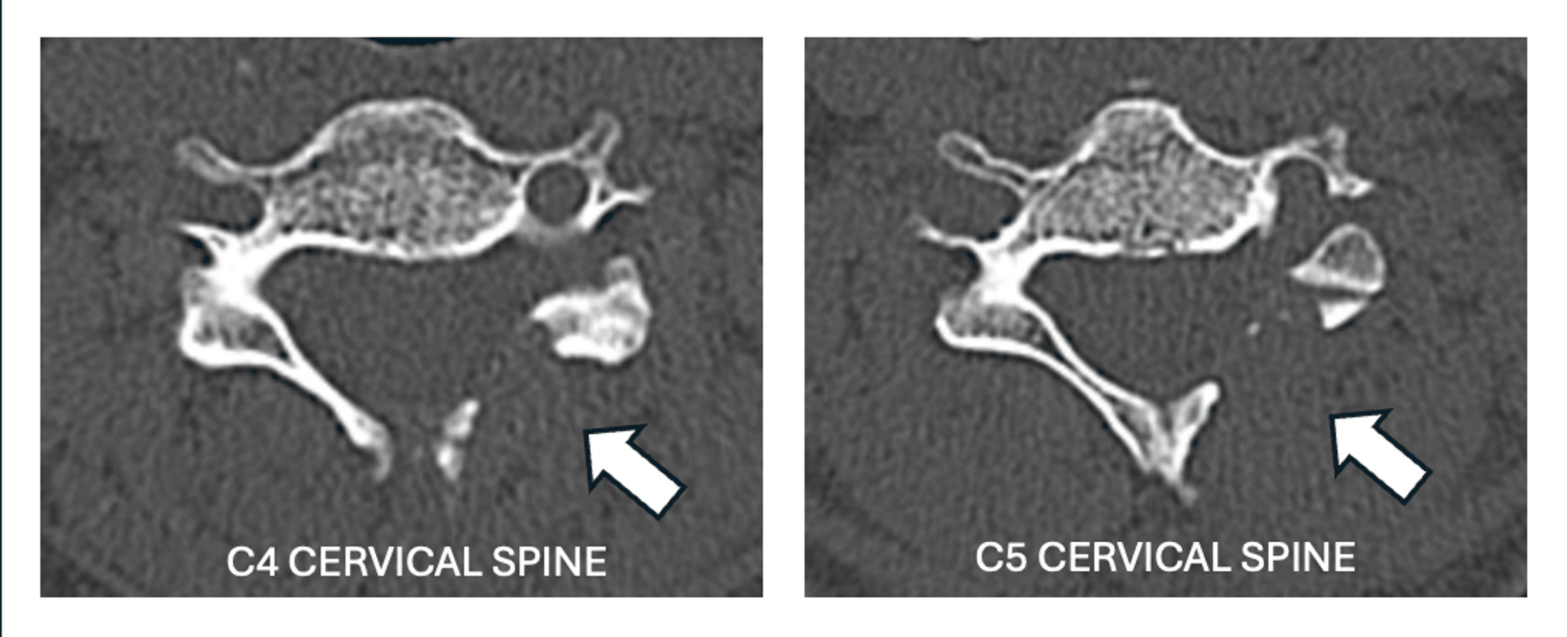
Postoperative CT scan in axial view at the C4 (left) and C5 cervical spine levels (right), demonstrating the left C4/5 and C5/6 neural foramina are capacious after the left C4/5 and C5/6 laminoforaminotomy procedure (arrows).
